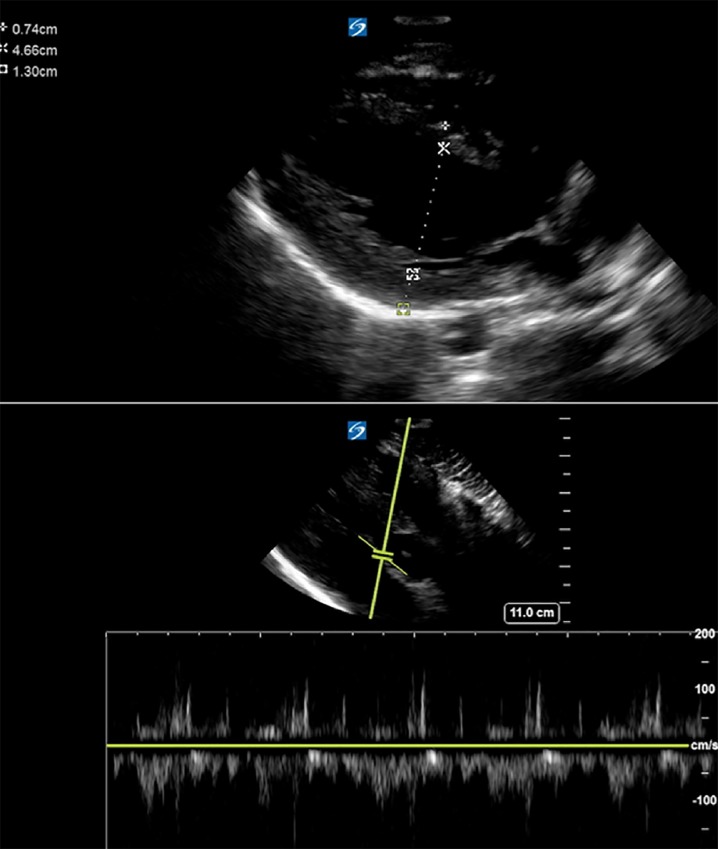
Ultrasound images of positive findings of point of care cardiac study. Posterior wall thickness of 1.3 centimeters coupled with the abnormal history and physical findings were concerning for this athlete.
